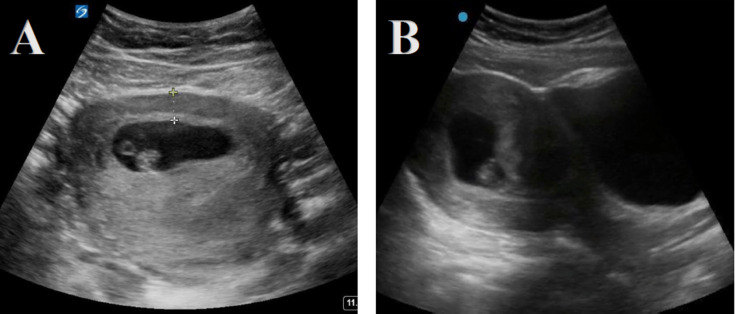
Point of care ultrasound (POCUS) demonstrating an intrauterine pregnancy (a gestational sac containing a yolk sac within the endometrium in 2 planes) with measurement of the myometrial mantle.
